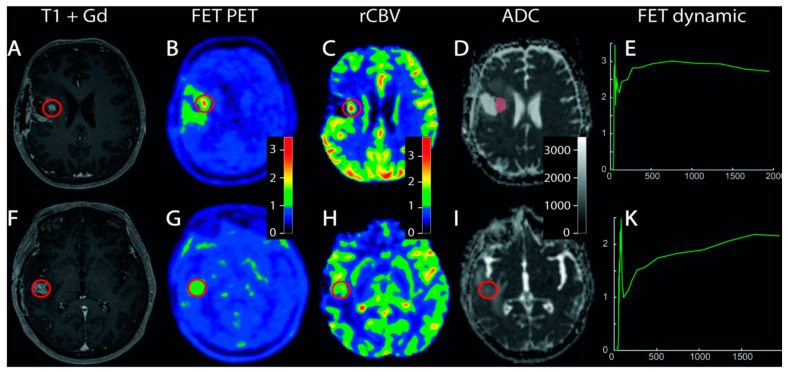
The increased uptake and early time to peak at [^18^F]FET PET/MRI allowed discriminating of tumour tissue in a patient with operated and irradiated anaplastic astrocytoma and a suspicious contrast-enhancing lesion appeared at MRI during follow-up (A–E). No significant uptake and late time to peak was observed in an area of contrast-enhancement at MRI in a patient with radio-chemo-treated glioblastoma, suggesting radiation necrosis (F–K). Reprinted/adapted with permission from Ref. [21]. Copyright © 2018 Elsevier B.V.
